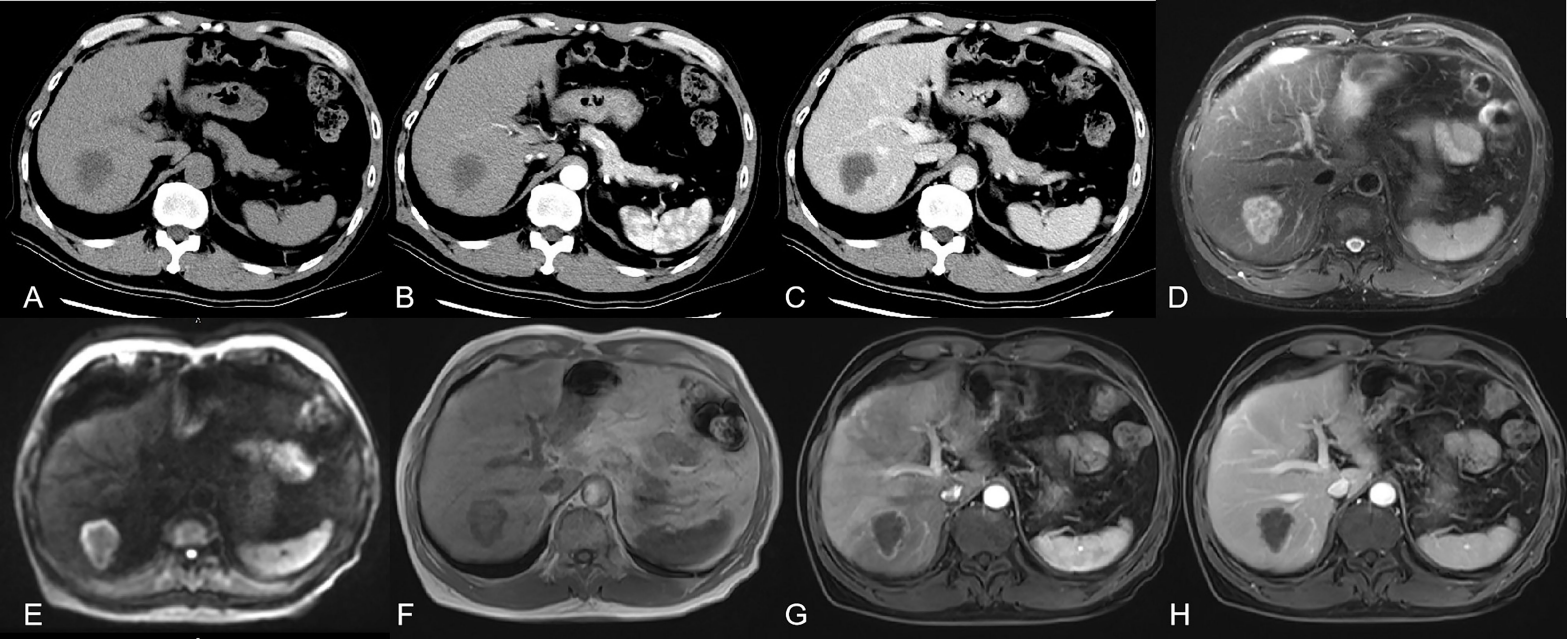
showed a 66 year old male patient with rectal cancer. (A) CT without contrast showed there was an irregular low-density focus in S7 segment of the liver, which could not be well diagnosed qualitatively. (B, C) CT with contrast showed there seemed to be slight enhancement at the edge of the lesion. CT imaging combined with the patient’s history could make an imaging diagnosis of suspected liver metastasis. (D-F) MRI without contrast could basically characterize the lesion as malignant. (G, H) with contrast could make a definite diagnosis of metastases.
